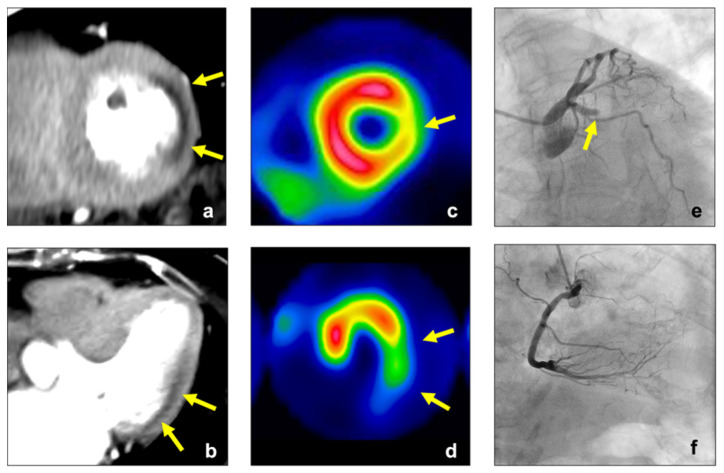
A 75-year-old woman with effort angina. Stress CTP image of the left ventricle showed a perfusion abnormality in the lateral wall (yellow arrow, (a,b)), and MPR in this lesion was lower than the cut-off value of MPR in this study (0.60 < cut-off value: 0.81). SPECT ((c,d), stress) showed a perfusion abnormality in the lateral wall (yellow arrow). ICA ((e), LCA, (f), RCA) revealed chronic total occlusion within the LCX. CTP, computed tomography perfusion; MPR, myocardial perfusion ratio; SPECT, single-photon emission computed tomography; ICA, invasive coronary angiography; LCA, left coronary artery; RCA, right coronary artery; LCX, left circumflex coronary artery.
